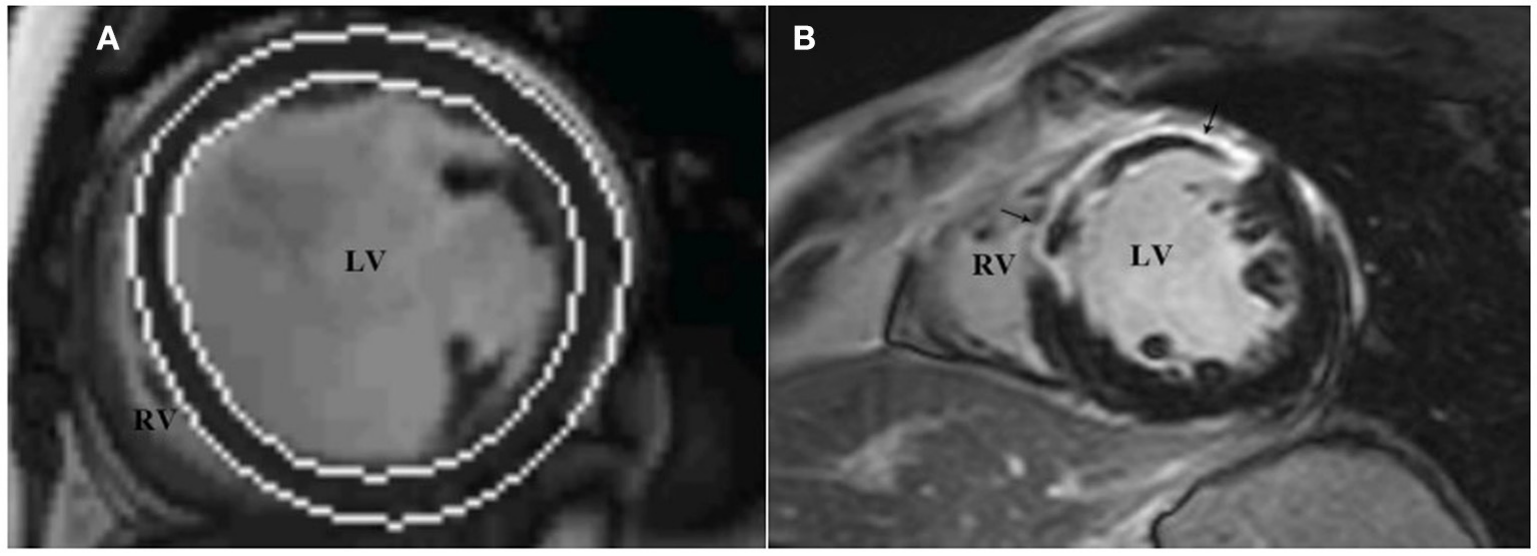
LV function and LGE analysis via cardiac magnetic resonance imaging. The analysis software automatically tracks the left ventricular endocardium and epicardium to measure left ventricular volume (A), and assesses the presence and distribution of left ventricular LGE through short-axis images (B), which show high signal intensity in the middle segment of the left ventricular septum, anterior wall. LGE, late gadolinium enhancement.
